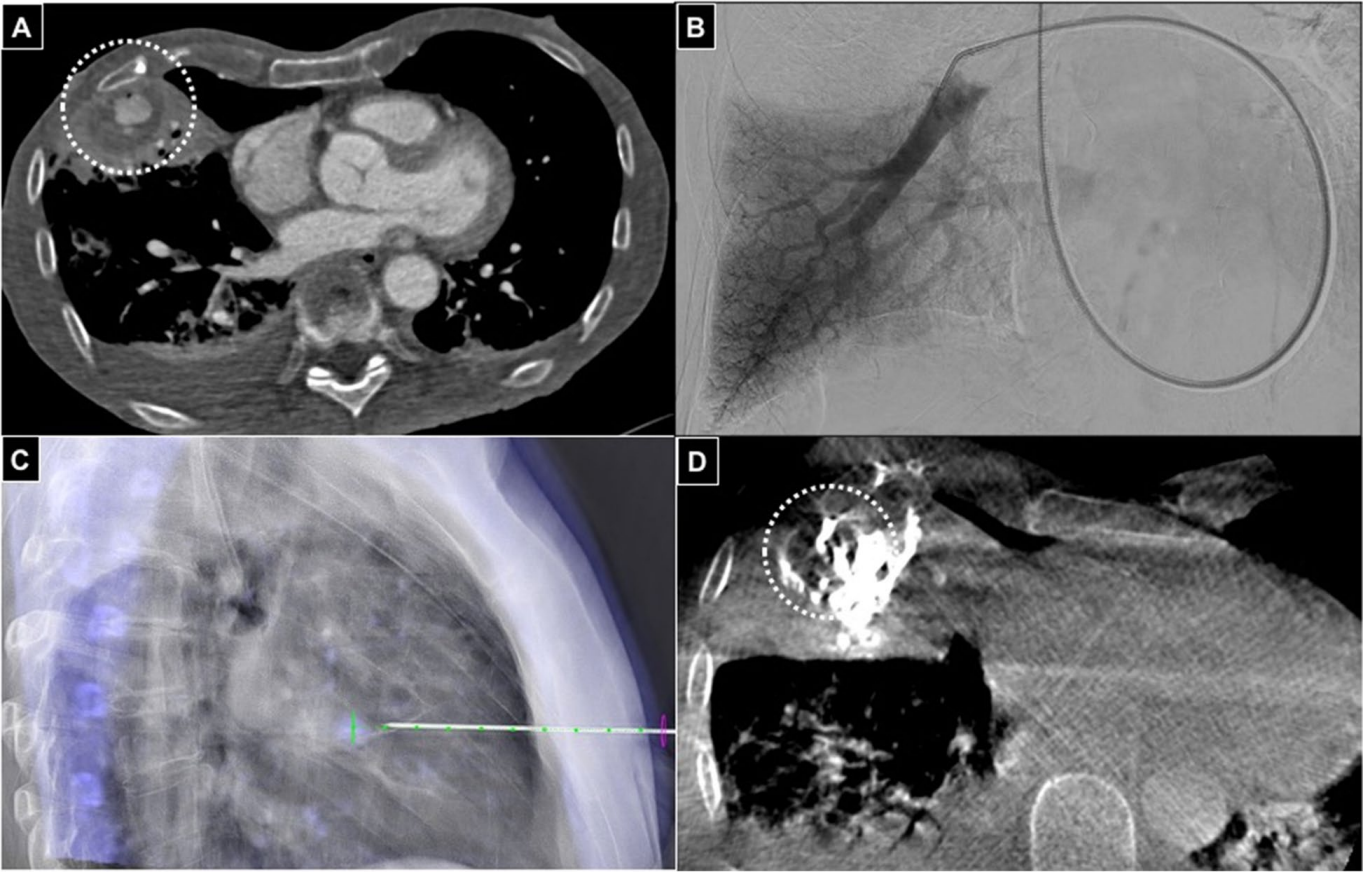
Sixty-four years old male with pneumonia. A Axial CT in arterial phase showing 25 mm pulmonary PA (white dotted circle) in the inferior lobe of the right lung. B\Right inferior lobe pulmonary DSA not depicting the lesion. C CBCT guided puncture using an 18G 15 cm needle with software assistance (Xpere Guide® - Philips). D Final CBCT showing the glue cast into the PA (white dotted circle)
